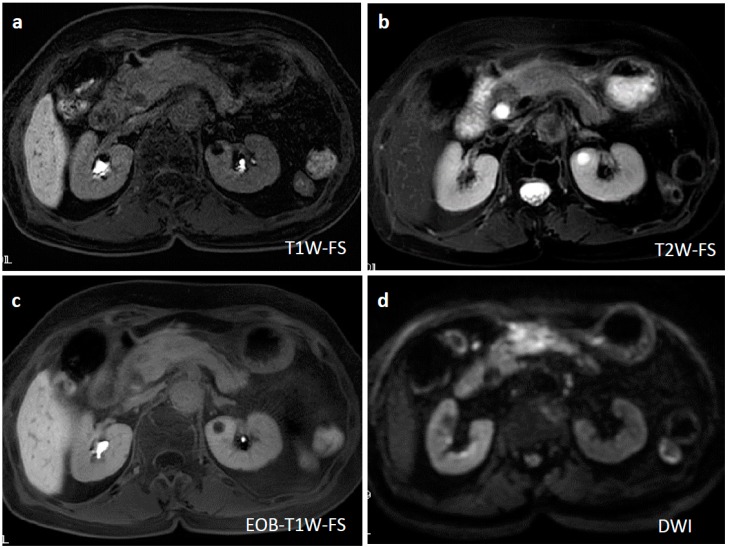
Magnetic resonance imaging (MRI) at two weeks after EUS-FNAB and three weeks after the initial CT. A T1-weighted MRI showed an iso-intensity signal (a), a T2-weighted image showed a faint low-intensity signal (b), a gadoxetate sodium-injected MRI demonstrated a slightly weak enhancement (c), and a diffusion-weighted MRI revealed heterogeneously repressed diffusion ability at the pancreatic lesion (d).
